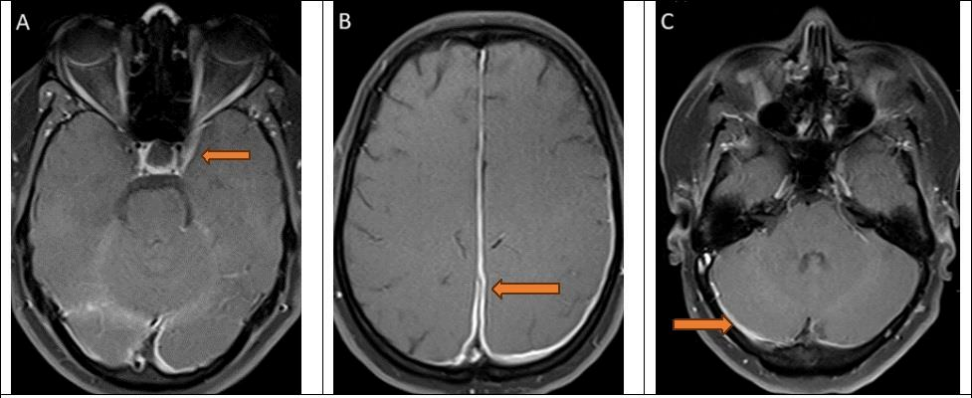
A 32-year-old woman with hypertrophic pachymeningitis presented with a headache. A) MRI Brain (axial section) shows meningeal enhancement in the left cavernous sinus extending into the left orbital apex. B) MRI Brain (axial section) shows meningeal enhancement in the interhemispheric fissure. C)MRI Brain (axial section) shows meningeal enhancement in the right cerebellar hemisphere
